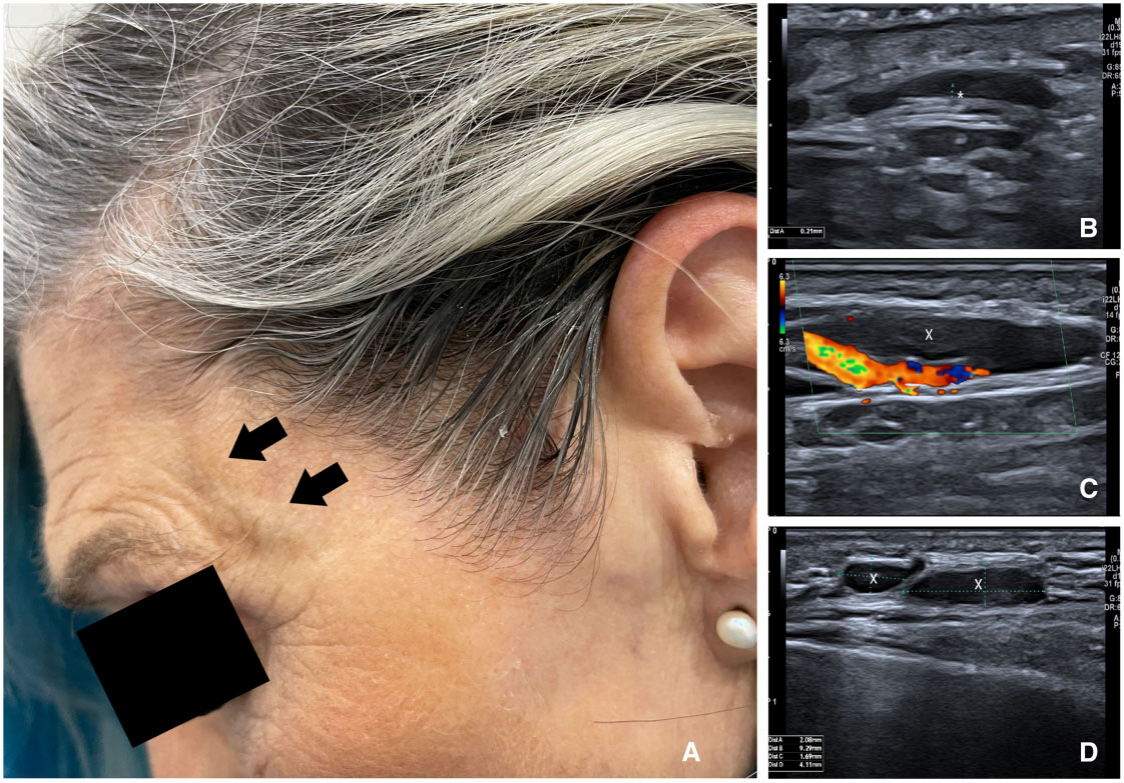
Patient with temporal swelling and its ultrasound evaluation. (A) Swelling in the left temporal area (arrows). (B) Ultrasound image of the left common superficial temporal artery, in transverse view, showing a normal intima–media complex with no halo sign (*). (C) Ultrasound image of a normal frontal branch of the left temporal artery, in transverse view, surrounded by a hypoechoic ovoid node (x). (D) Ultrasound image of two hypoechoic nodes with fatty hilum suggestive of lymph nodes
